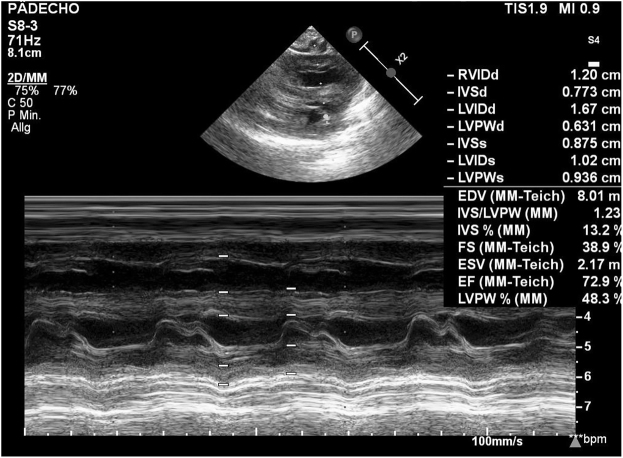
Neonatal echocardiography.
